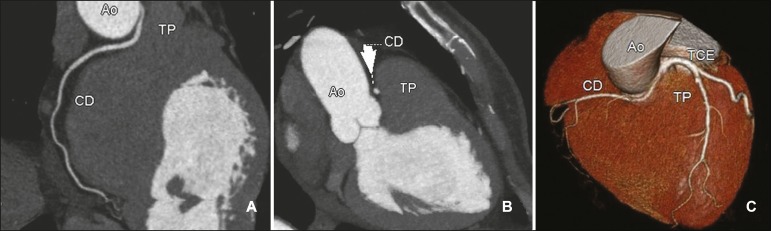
Contrast-enhanced CT. Oblique reconstructions (A,B) and a
